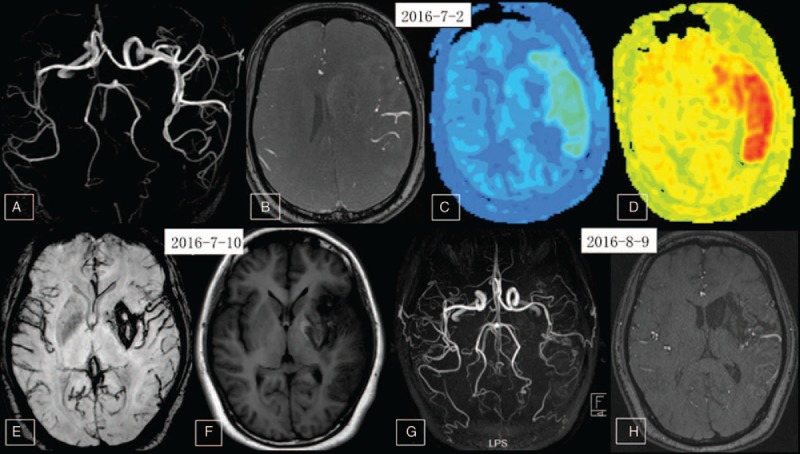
Follow-up MR angiography (MRA), arterial spin labeling (ASL), and susceptibility weighted imaging (SWI) results. (A) Time of flow MRA (TOF-MRA) showing marked left MCA dilatation at 9 days after disease onset; (B) TOF-MRA source image also indicated left MCA dilatation; (C, D) ASL perfusion image showed left MCA distribution hyperperfusion; (E, F) repeated MR image at about 2 weeks post-onset (C: SWI, F: T1-weighted image) showing large infarction of the left MCA distribution with HT and brain edema. Results show left infarction lesion with reduced HT and brain edema; (G,F) Repeated MRA and source image showing that the dilated left MCA recovered to normal but artery branch number was lower than the right MCA.ASL = arterial spin labeling, HT = hemorrhagic transformation, MCA = middle cerebral artery, MRA = MR angiography, TOF-MRA = time of flow MRA.
